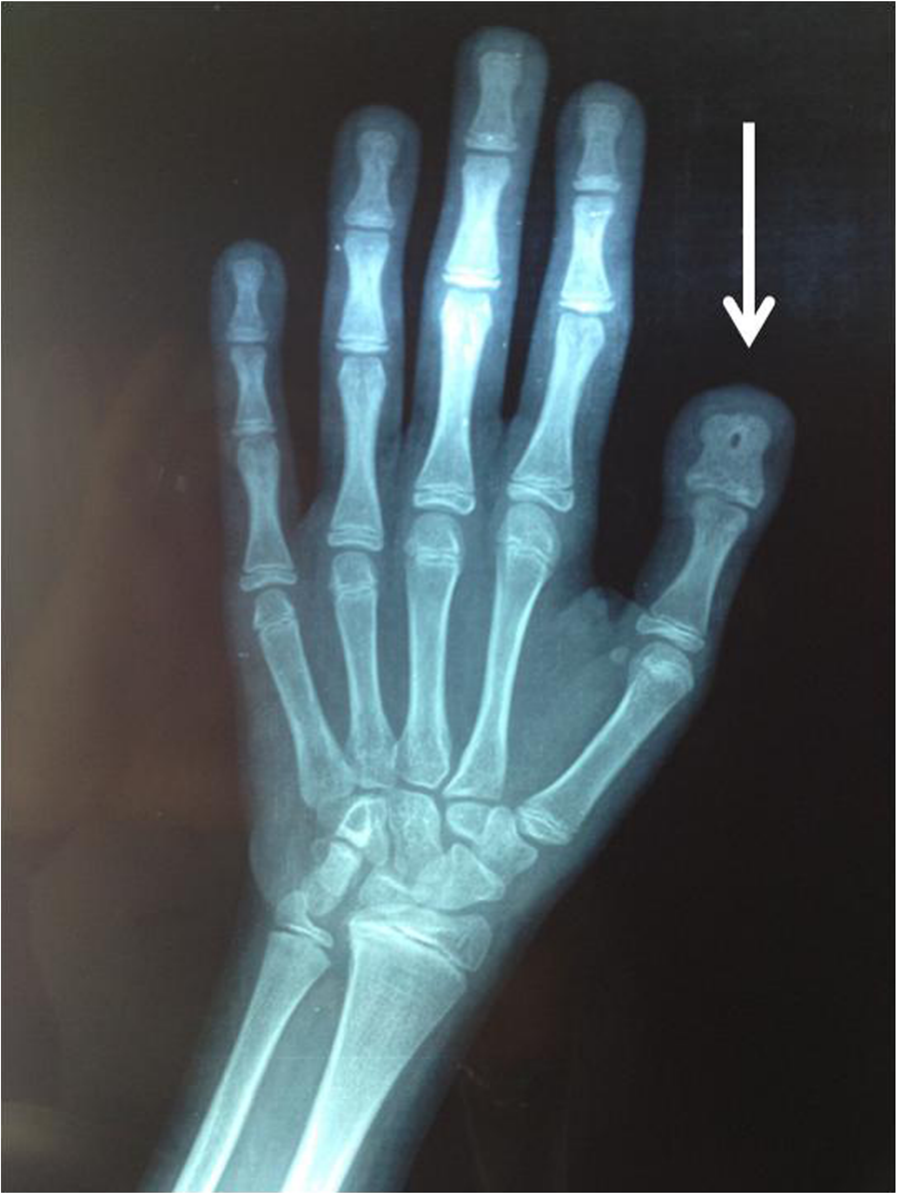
This hand wrist radiograph was made when she was 14 years old. The arrow shows a duplication of terminal phalanx of the thumb.
